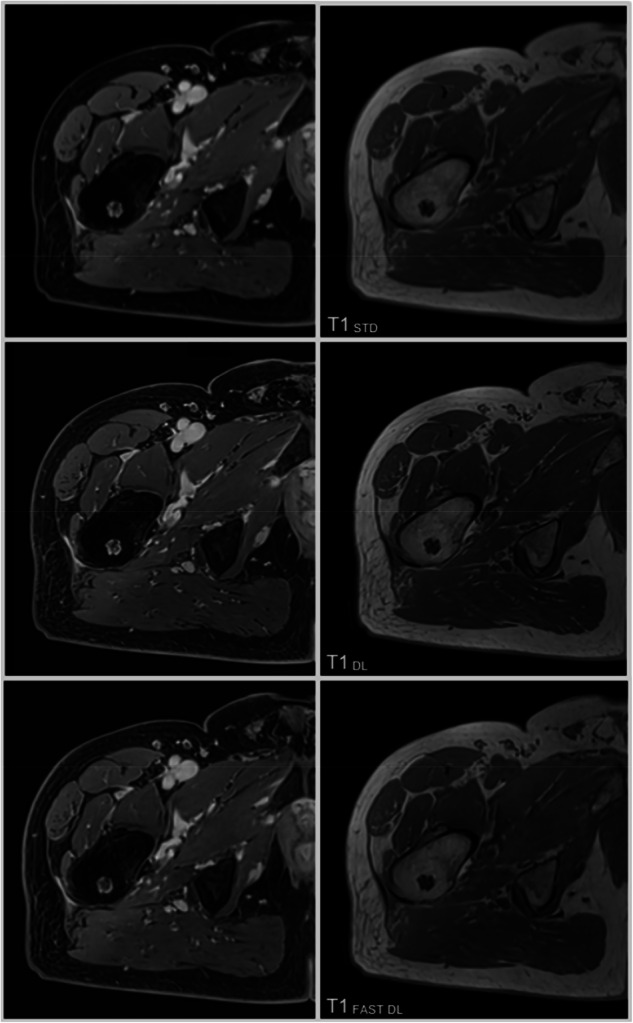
A benign bone lesion, an enchondroma, of the right proximal femur of a 62-year-old patient with a PSA of 6.8 ng/mL and suspected prostate cancer. T1-weighted sequences with contrast- enhanced (CE) water-only images on the right and non-contrast in-phase images on the left are shown. Standard sequence (T1_STD_); research DL-accelerated T1-weighted sequences (T1_DL_ with 45-s and T1_DL FAST_ with 15-s acquisition time, respectively)
